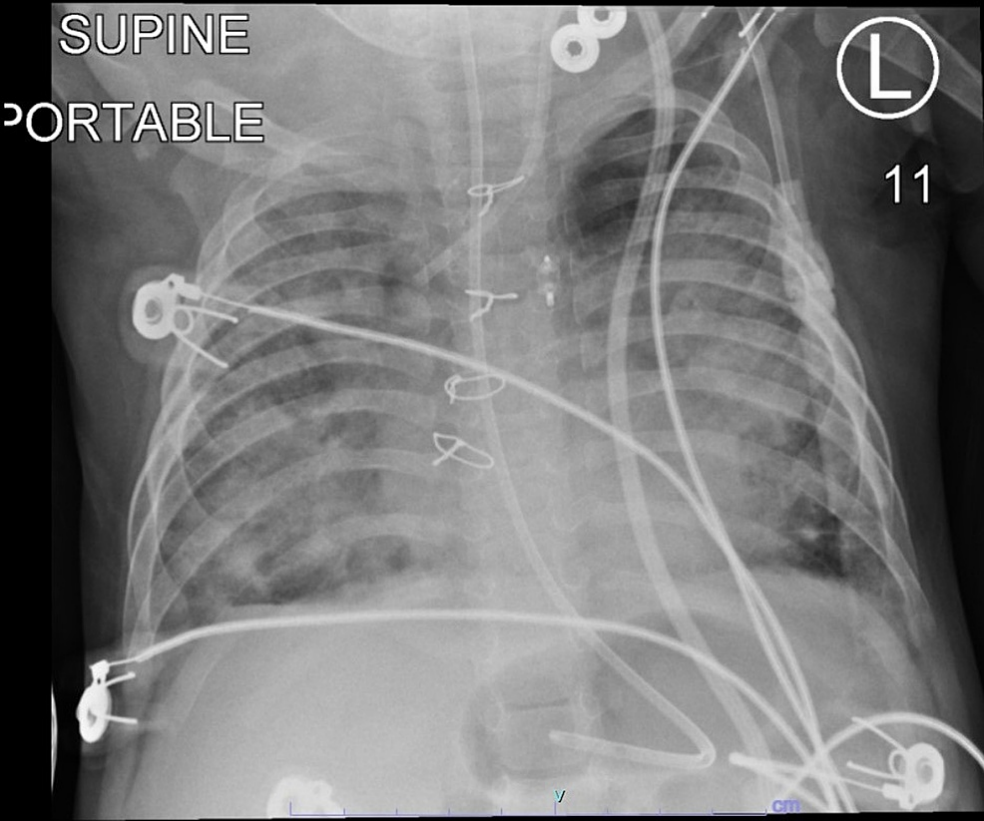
Chest X-ray demonstrating extensive consolidation superimposed on chronic interstitial markings secondary to bronchopulmonary dysplasia.
